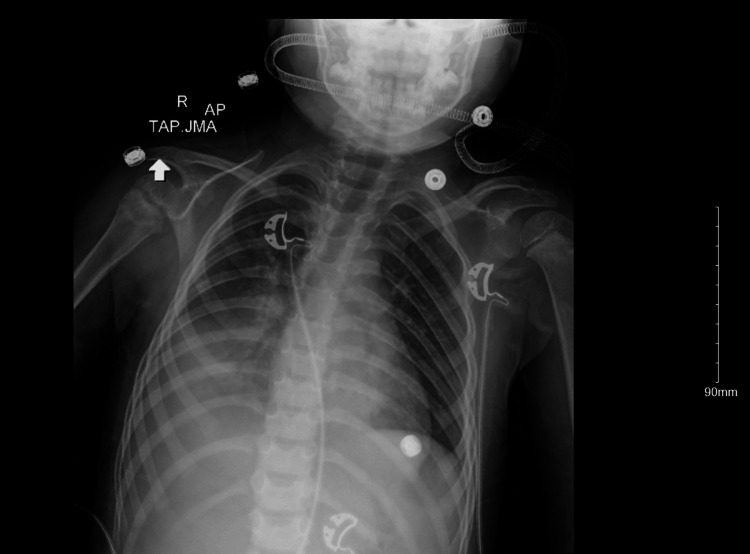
AP CXR at initial presentation showing basilar opacifications superimposed on moderate peribronchial wall thickening. No significant pleural process. Normal cardiac silhouette. AP: anterior-posterior view; CXR: chest X-ray
